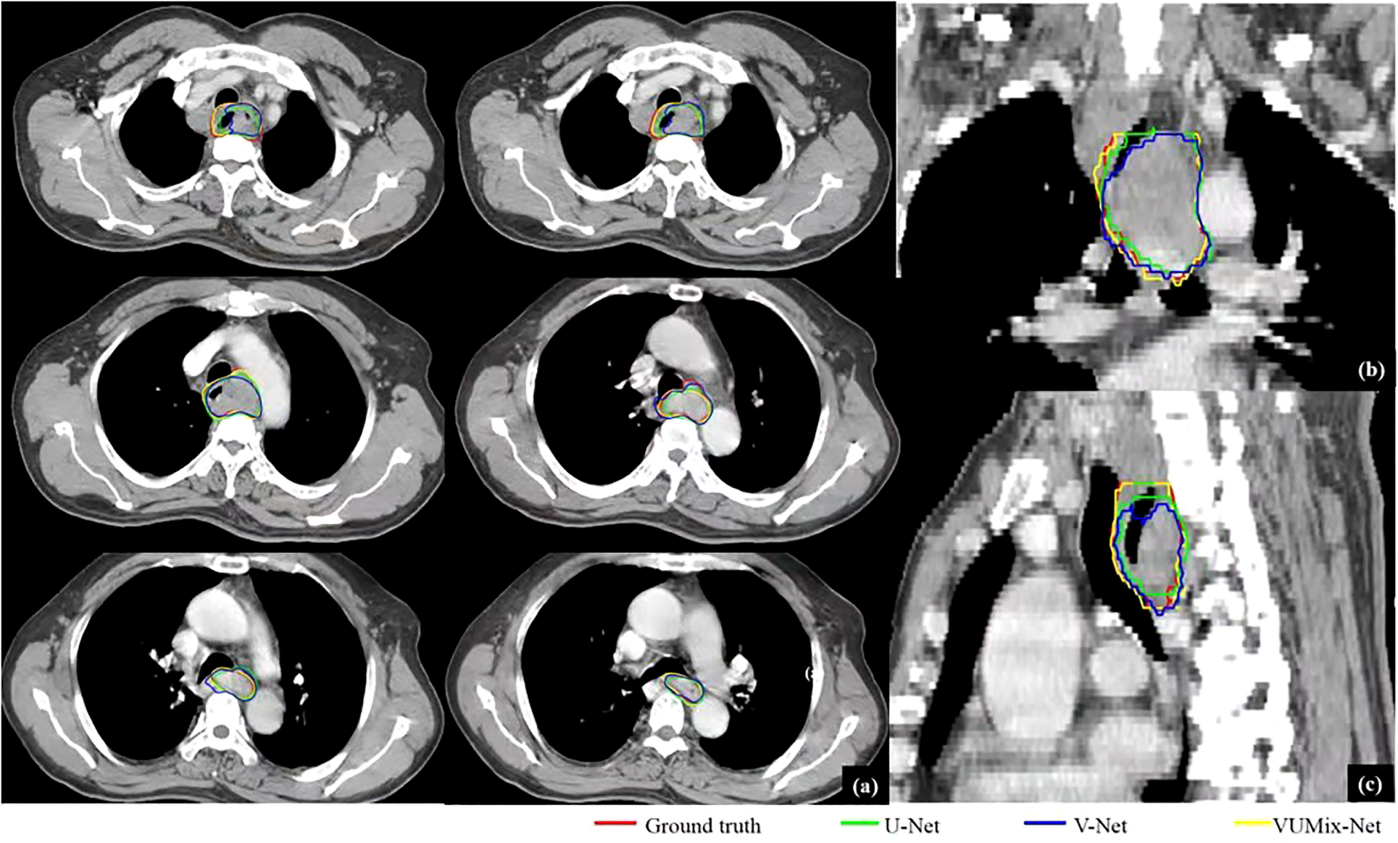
GTV delineations for a patient predicted from U-Net, V-Net, and VUMix-Net. (A) GTV on six transversal planes, (B) GTV on a coronal plane, (C) GTV on a sagittal plane. The CT slices were scanned from an upper esophageal cancer patient.
